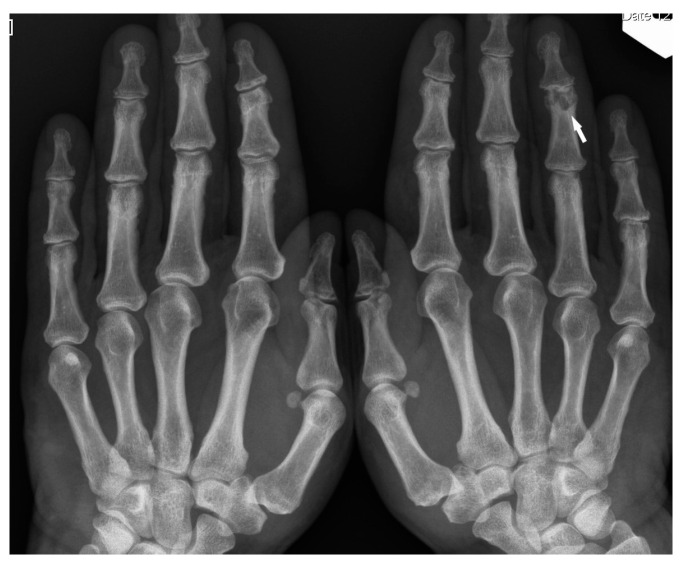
X-ray of the hands, PA. Unilateral osteolytic-cystic lesions in the subchondral layer of the middle phalanx of the 4th finger of the left hand (white arrow).
